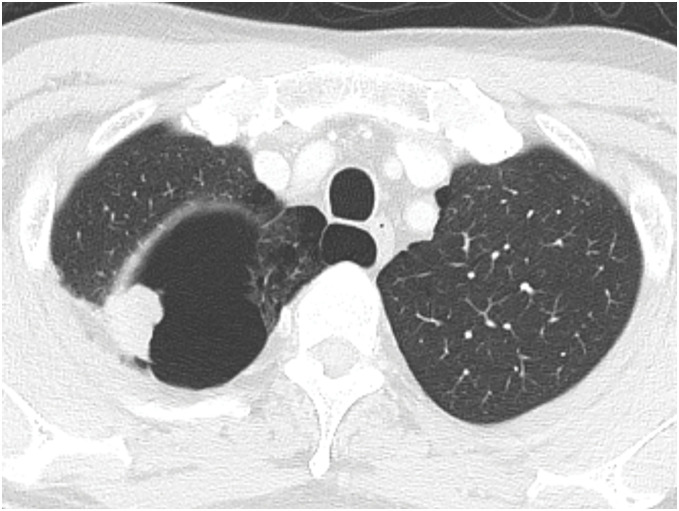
Preoperative chest CT image showing a solid nodule measuring 2.2 cm in the right upper lobe’s apical segment.
